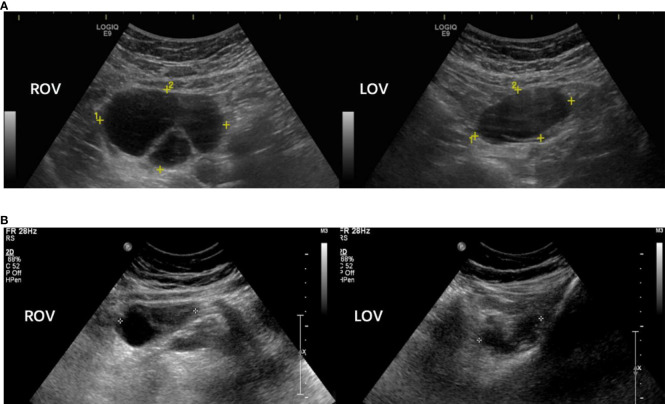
Sonographic pictures of a 18 year-old p17OHD patient’s multi-locular ovaries before (A) and after (B) combined oral contraceptive treatment (Case 2). Note: Rov, right ovary; Lov, left ovary.
